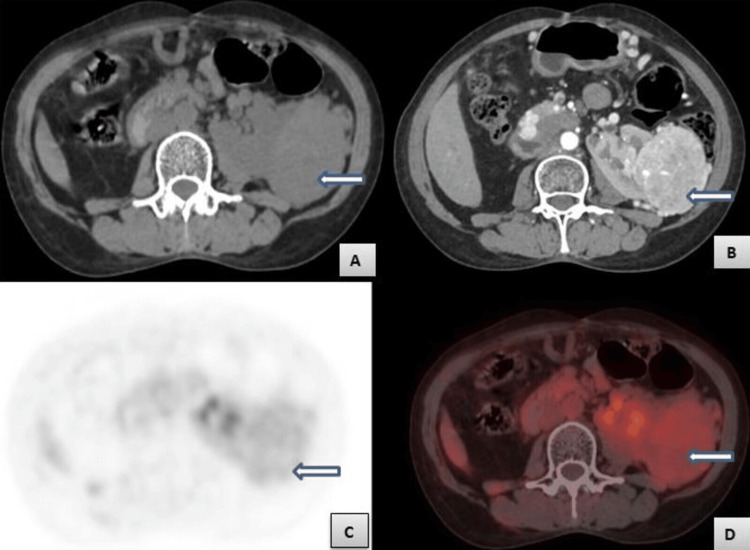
Axial computed tomography (CT) and positron emission tomography-computed tomography (PET-CT) at the level of kidney Figure 2A - Axial pre-contrast CT image showing tissue density mass in the left renal moiety (white arrow). Figure 2B - Axial post-contrast CT image in arterial phase showing enhancing mass in the left renal moiety (white arrow). Figures 2C-2D - Axial PET and PET-CT images showing diffuse uptake of FDG by the lesion in the left renal moiety (white arrows) with a standardized uptake value of 4.1. FDG: fluorodeoxyglucose
